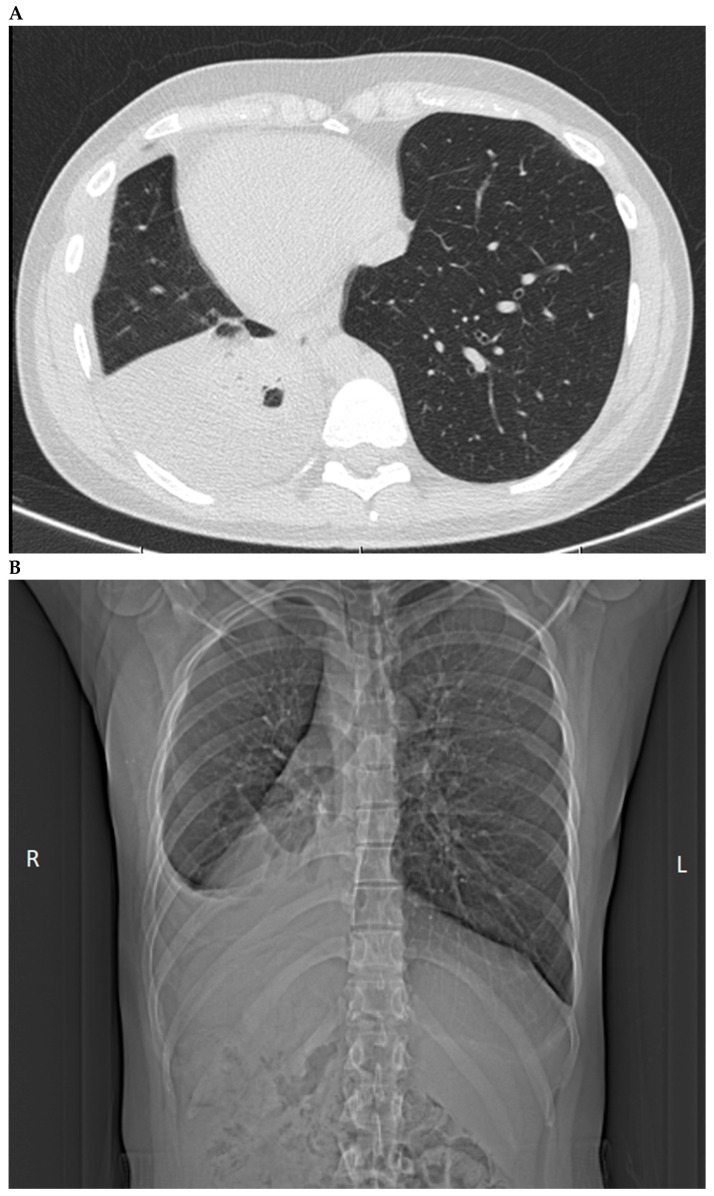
(A,B) Chest CT images showing a significant presence of fluid in the right lung, taken during the second hospitalization. There is also evidence of situs inversus in the patient (detailed description in Appendix B).
